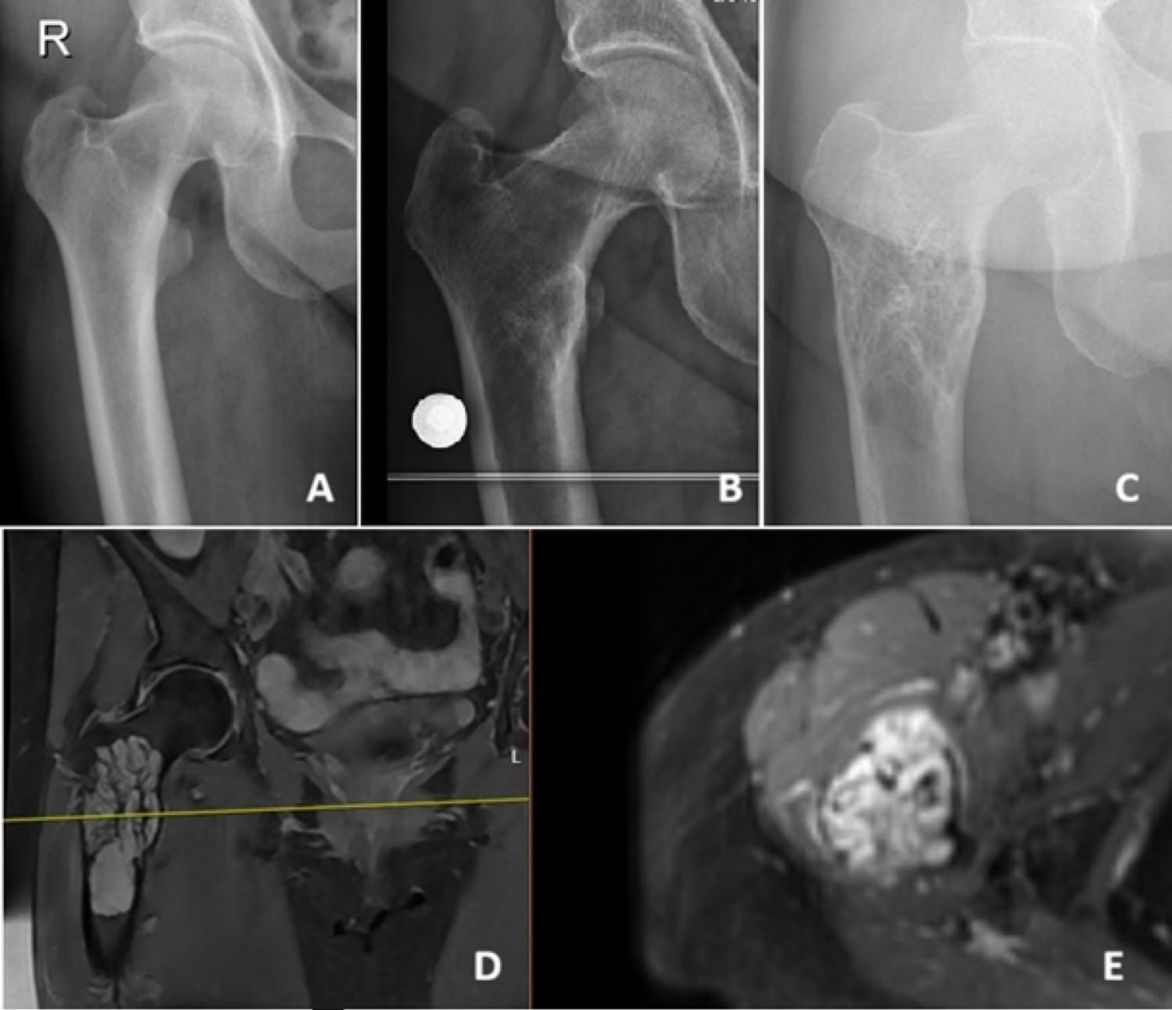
Epithelioid hemangioma in the proximal femur. (A) Radiograph of the right hip 10 years before presentation, no detectable lesion. (B) Radiograph of the right hip 2 years before presentation, small medullary lesion adjacent to the lesser trochanter. (C) Radiograph at presentation, showing marked lesion growth. (D) Coronal MRI at presentation, demonstrating a medullary lesion filling the intertrochanteric region and extending below the lesser trochanter. (E) Axial MRI slice shows surrounding reactive changes
